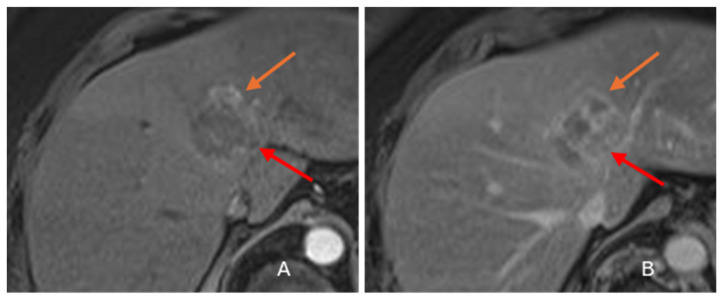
Axial gadolinium-enhanced MRI images in an 82-year-old female with F3 to 4 fibrosis and a pathologically proven cHCC-CC on resection. A 4 cm cHCC-CC in segment 4A shows overlapping features of both HCC and ICC with two distinct foci of differing enhancement within the tumor. A 1.2 cm focus at the 11 to 1 o’clock position (orange arrows) shows LR5 features, including heterogeneous arterial enhancement (A), followed by delayed phase washout (B). A 2.4 cm focus at the 2 to 5 o’clock position (red arrows) shows LR M features, including peripheral arterial enhancement (A), followed by delayed central progression (B).
